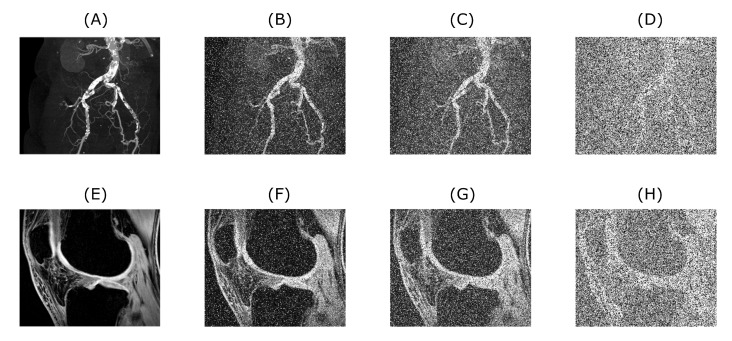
Upper row is showing a CT scan of blood vessel calcification with the native level of noise (A) used as an input for algorithm testing. Images (B–D) are showing the original image with added artificial noise for 0.1, 0.2 and 0.5 level (in fact the noise consists of a sum of three noise types, in which the number means a mean value for additive white Gaussian noise and density for salt and pepper noise, speckle noise is kept on a constant default value used in MATLAB), respectively. Lower row shows the example of MRI scan of knee cartilage (E used as an input), again with different consecutive noise levels of corresponding magnitude (F–H).
